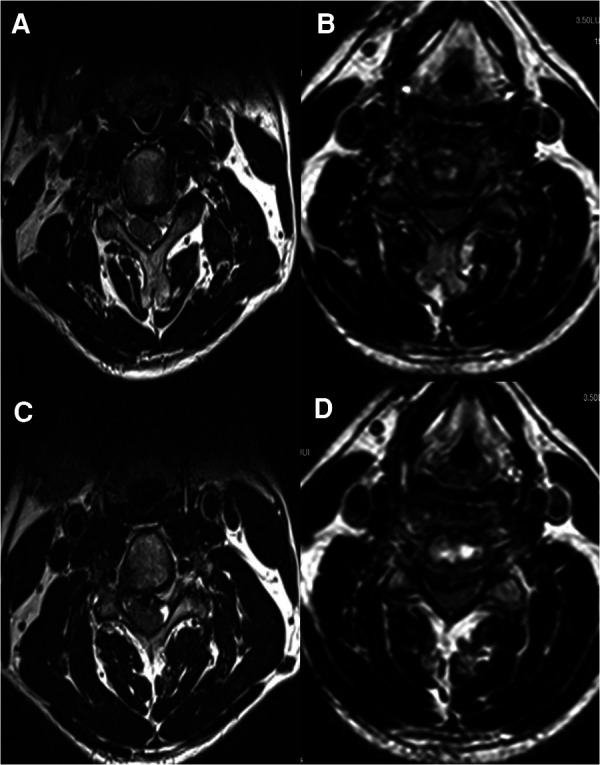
(A) Preoperative C3/4 MRI cross-section. (B) Postoperative C3/4 MRI cross-section. (C) Preoperative C4/5 MRI cross-section. (D) Postoperative C4/5 MRI cross-section.
